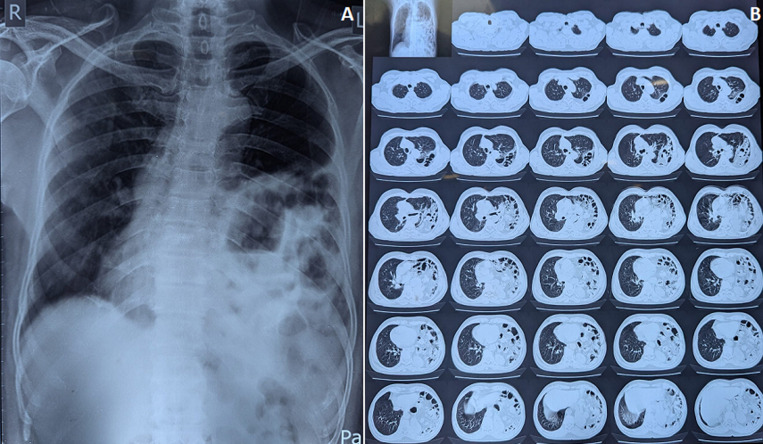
A) chest X-ray showing herniation of the stomach and small bowel loops into the left thoracic cavity with rightward mediastinal shift; B) high-resolution computed tomography of the thorax showing ~5.8 cm posterolateral defect in the left hemidiaphragm
